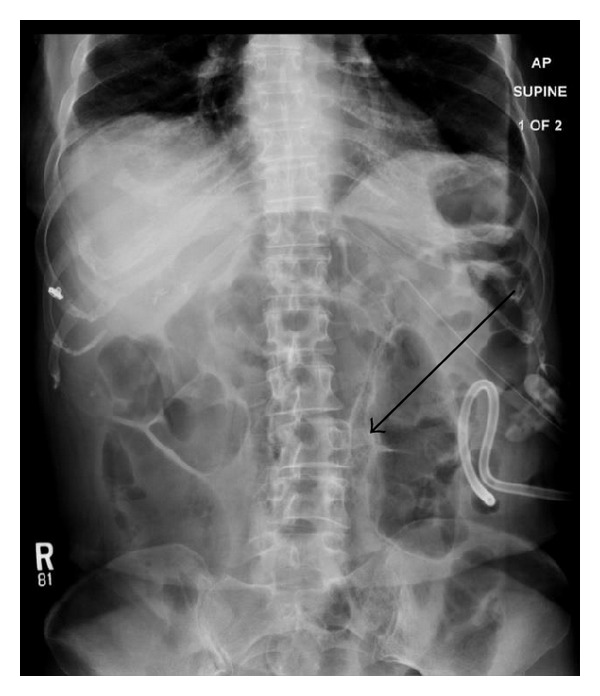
Abdominal radiograph demonstrates gastrostomy tube and jejunostomy tube in the left upper quadrant of the abdomen. Multiple small round lucencies are seen in the wall of loops of small bowel in the midabdomen (arrows).
